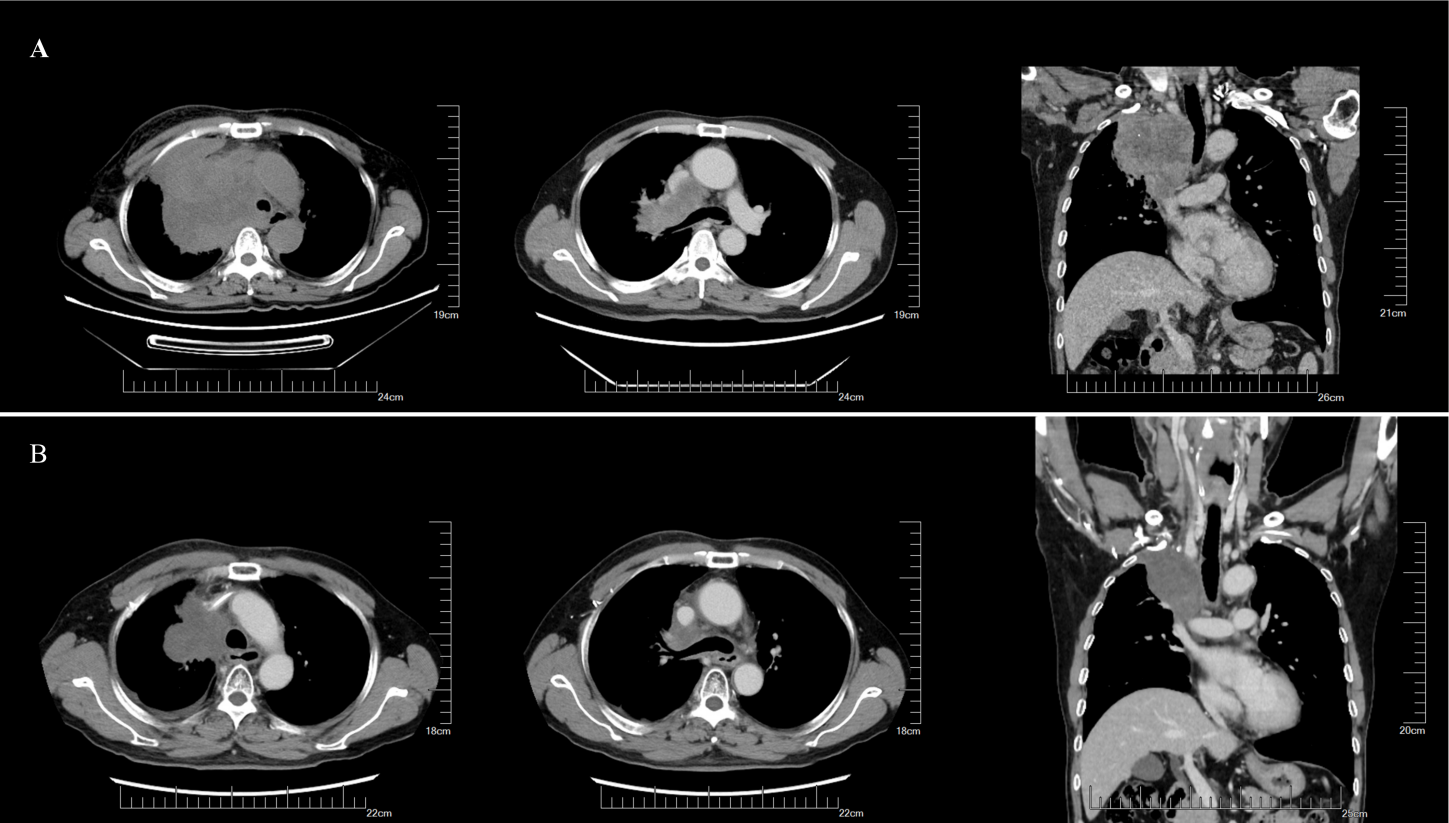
Efficacy of initial radiotherapy. (A) On initial presentation, enhanced CT revealed a mass in the right upper hilar region and right upper lobe with surrounding obstructive pneumonia, as well as tumor thrombus formation in the right brachiocephalic vein and azygos vein. (B) After radiotherapy, follow-up imaging showed significant reduction in the hilar mass.
